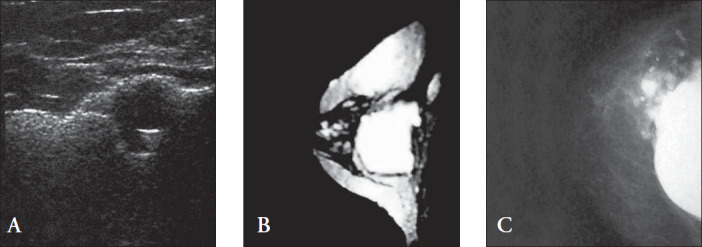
(A) Longitudinal ultrasonogram in the upper lateral quadrant region of the right breast showing an echolucent globule adjacent to snowstorm appearance. (B) Sagittal fast spin echo magnetic resonance image showing localized rupture of the implant in the right breast. (C) Craniocaudal view mammogram showing abnormal implant, thereby demonstrating rupture and extravasation of free silicone into the breast.
